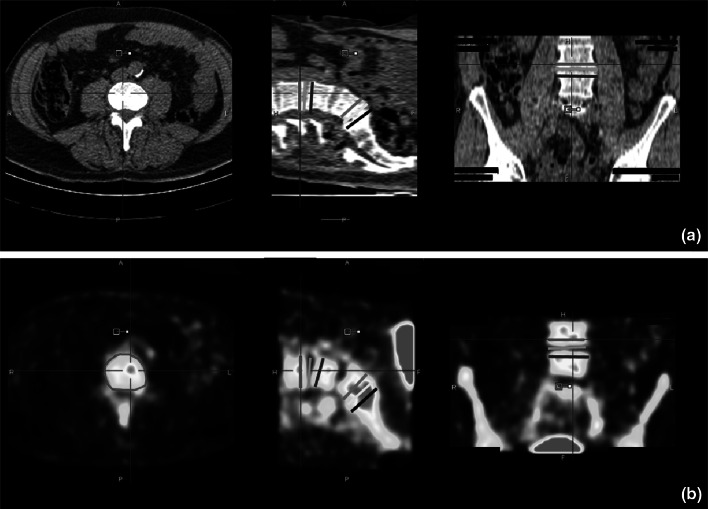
ROI definition. a An axial, sagittal and coronal CT image (from *left* to *right*) of the lower spine after PLIF. Three ROIs were drawn in the operated segment: the lower endplate of the cranial vertebra (*pink*), the intervertebral disc (*yellow*), the upper endplate of the caudal vertebra (*black*). The same three ROIs were drawn in a normal reference segment 2 levels above the operated segment (*red*, *green* and *blue*, respectively). b The six ROIs were transferred to the co-registered PET image (axial, sagittal and coronal views from *left* to *right*)
